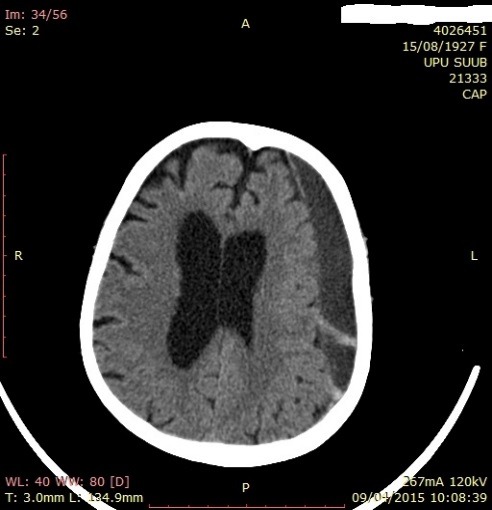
CT scan at readmission after 30 days in patient P.M. of 87 years old; left brain hemisphere chronic subdural haematoma
